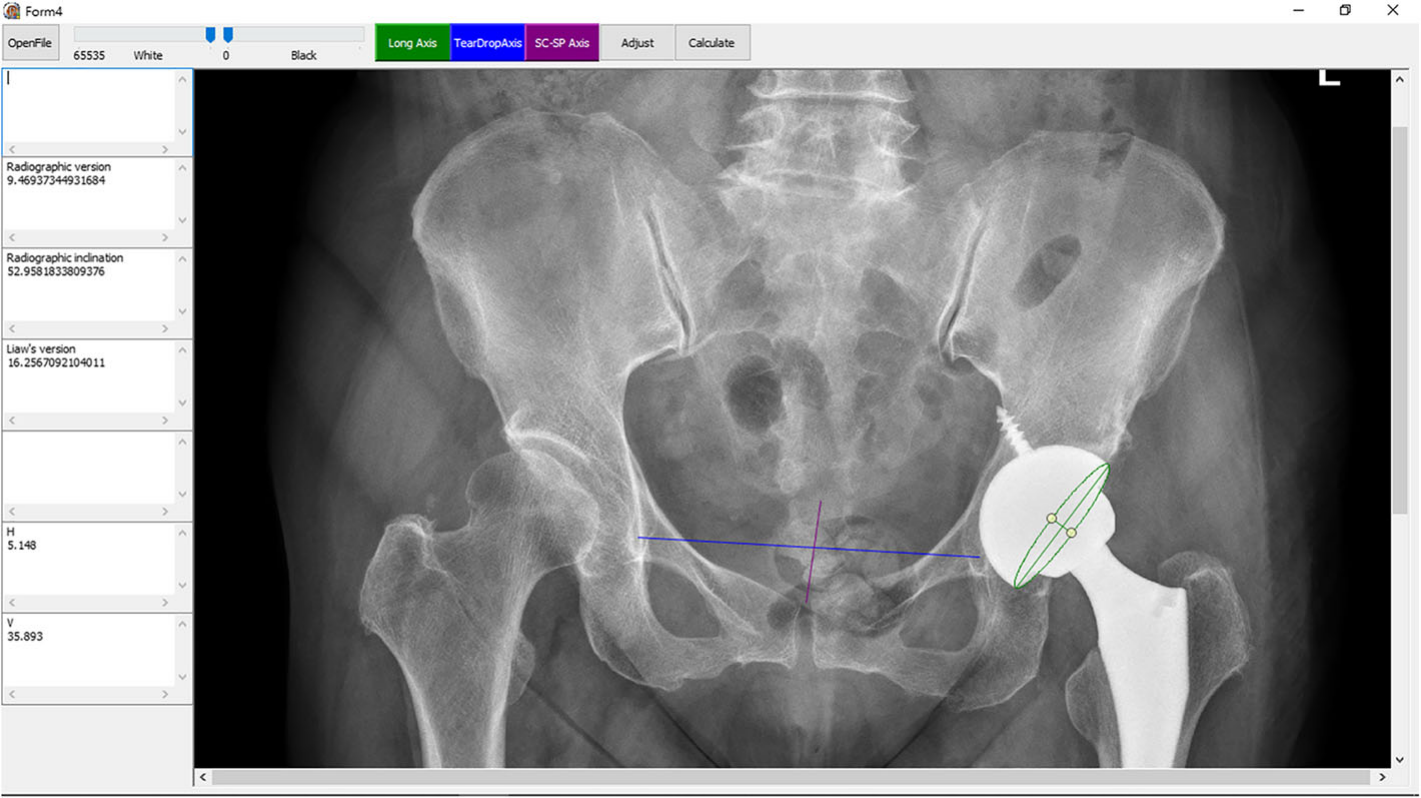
Ellipse method to measure the cup anteversion. The green line represents the cup margin of the cup, which is drawn by adapting the major and minor axes. The purple line represents the radiographic pelvic axis. The blue line represents the trans-teardrop line
